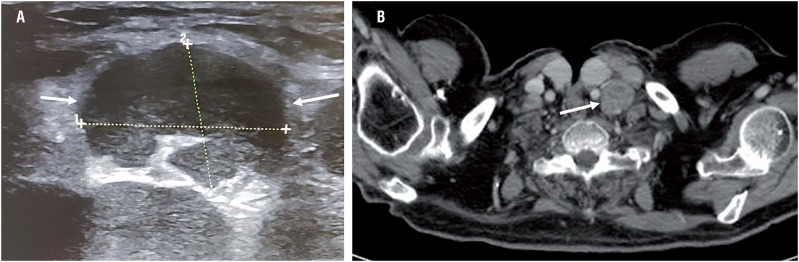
(A) Ultrasound of the left supraclavicular region and (B) contrast-enhanced computed tomography scan showing enlarged left supraclavicular LNs (arrows).
